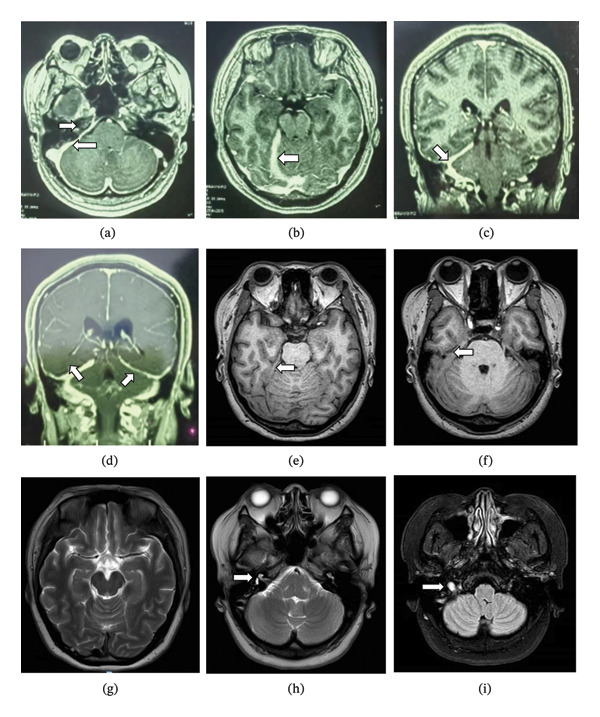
Cranial MRI. Images (a–c) show scans obtained 1 year earlier, and image (d) was acquired 4 months prior. Axial scans (a, b) and coronal scans (c, d) demonstrate marked dural and tentorial thickening in the right posterior cranial fossa, with abnormal signal intensity in the right inner ear. Images (e–i), obtained in August 2025, demonstrate thickening of the right cerebellar tentorium on T1‐weighted imaging (e, f) and abnormal hyperintensity of the right inner ear on T2‐weighted (g, h) and FLAIR (i) sequences.
